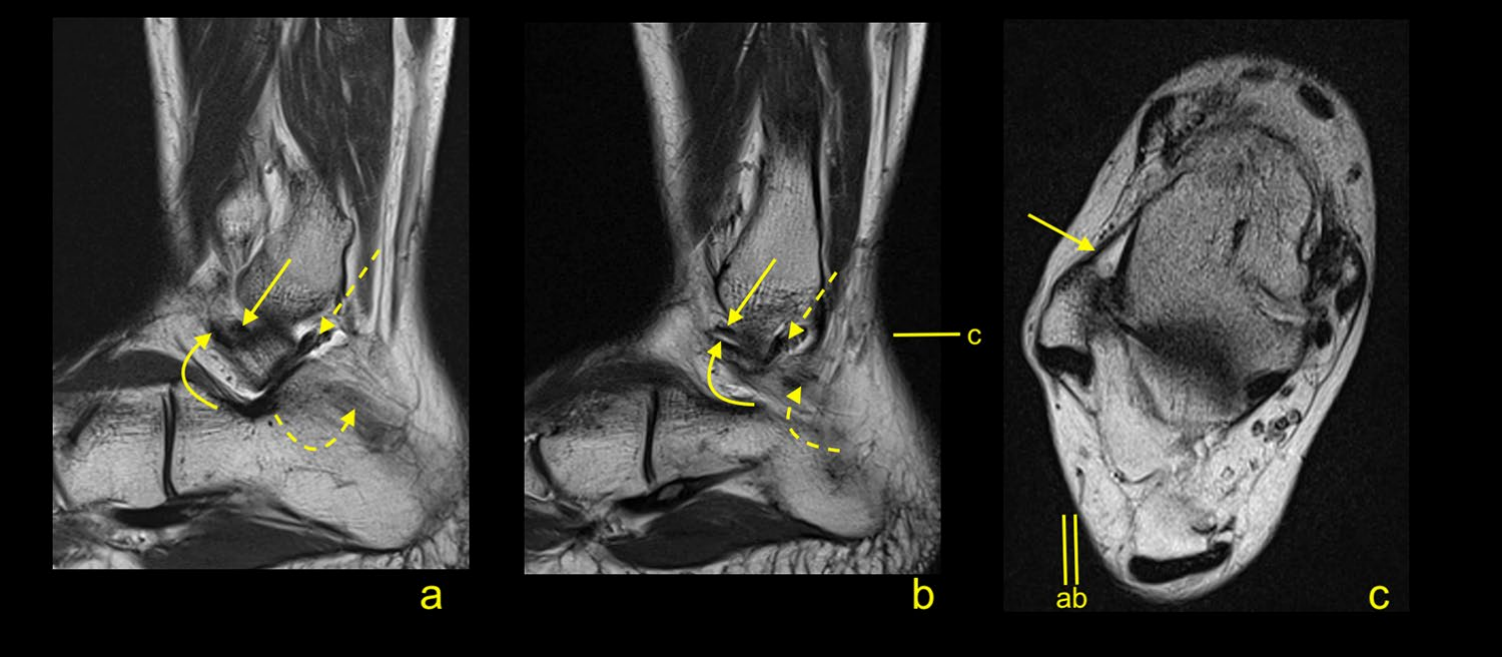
A 45-yeard-old patient with suspicion of a soft tissue tumor, which was not confirmed on the MRI. The double fascicular ATFL, two fascicles with the same diameter. Sagittal section (a,b) and transverse section (c). The S-ATFL (straight arrow), the I-ATFL (curved arrow), the PTFL (dashed arrow), and the CFL (curved dashed arrow). Levels of cross-sections are labeled on figures (b,c).
